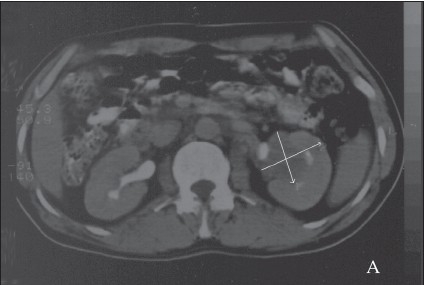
A CT-scan shows a homogenous low-density area involving the left renal sinus that shows distortion and displacement of the pelvicalyceal system without hydronephrosis (arrows demonstrates).
